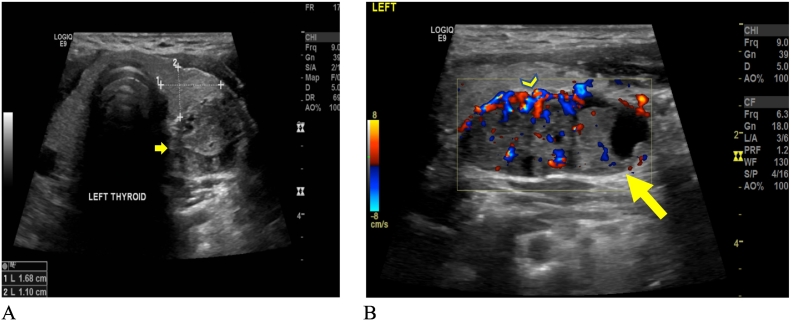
Thyroid ultrasound. Thyroid Ultrasound (A-B) showing well-defined, mildly lobulated, solid and cystic, predominantly solid, with mixed echogenicity, oval lesion (yellow arrow) with increased peripheral vascularity (arrowhead) lying posterior to the left lobe of the thyroid gland. (For interpretation of the references to color in this figure legend, the reader is referred to the web version of this article.)
